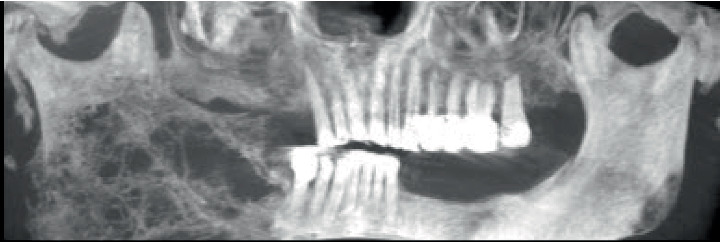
Reconstructed panoramic view generated from OnDemand3D App software (Cybermed Inc., Seoul, Korea) after reconstructing the computed tomography planes.
